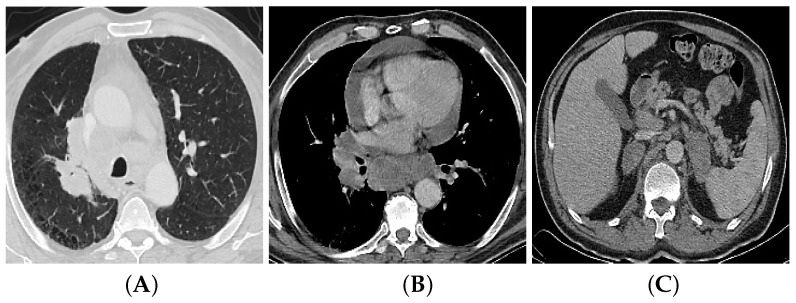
A 64-year-old male cigarette smoker with bronchogenic adenocarcinoma. Axial CT image (A) reveals spiculated right upper lung lobe mass (4.5×2.5 cm). Axial CT image at distal level (B) reveals ipsilateral hilar, mediastinal LN with central necrosis, and pericardial effusion. Axial CT image of upper abdomen (C) reveals bilateral metastatic suprarenal masses.
